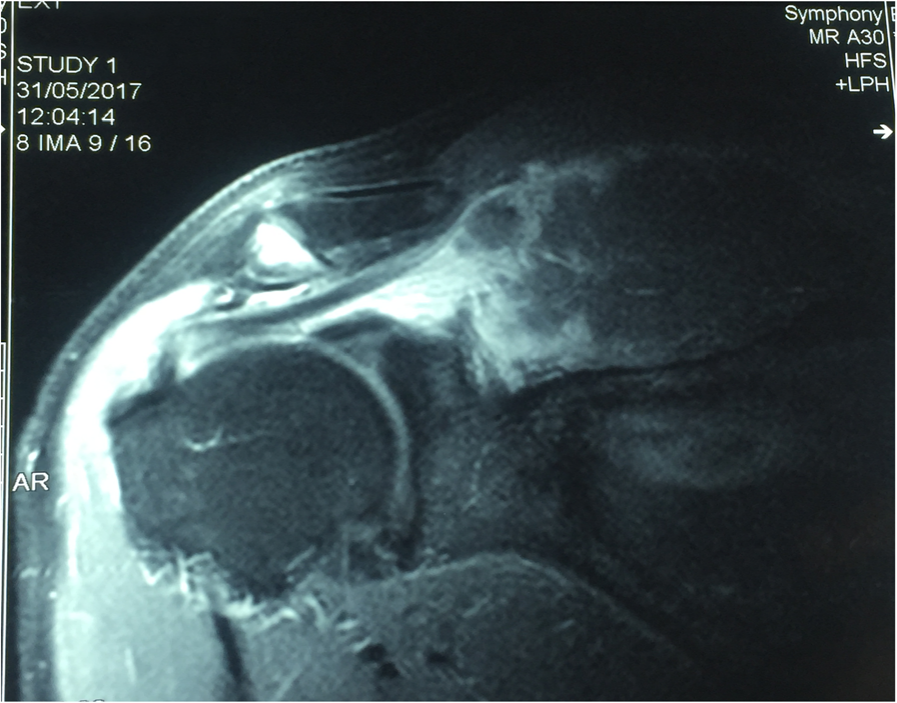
Magnetic resonance imaging of the scapular region with malignant tumor process centered on the supraspinous and infraspinous muscles
